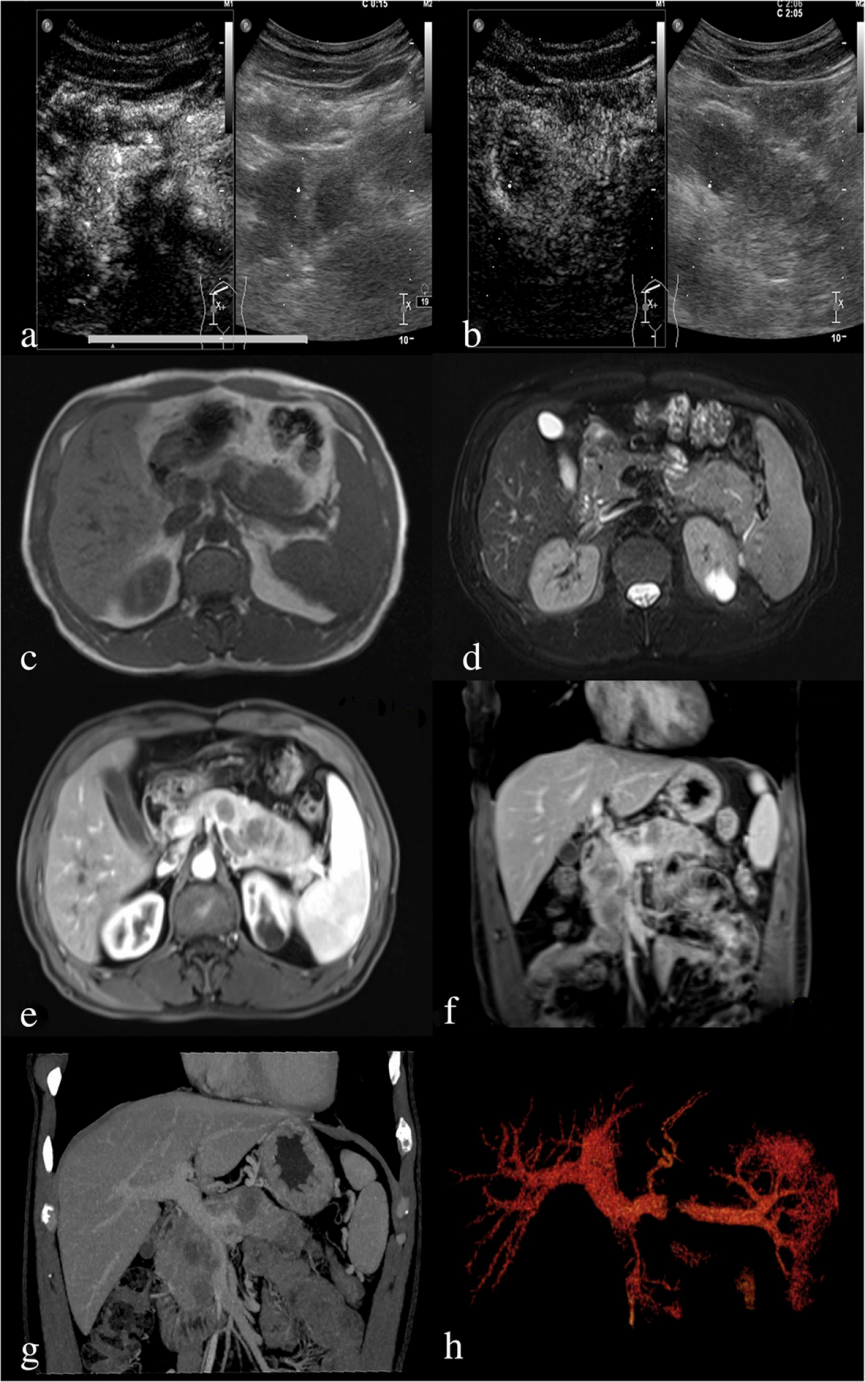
Contrast-enhanced ultrasonography (a, b), MRI (c, d, e, f) and CT (g) manifestation of pancreatic melanoma. Three-dimensional vascular reconstruction of portal vein system (h)
